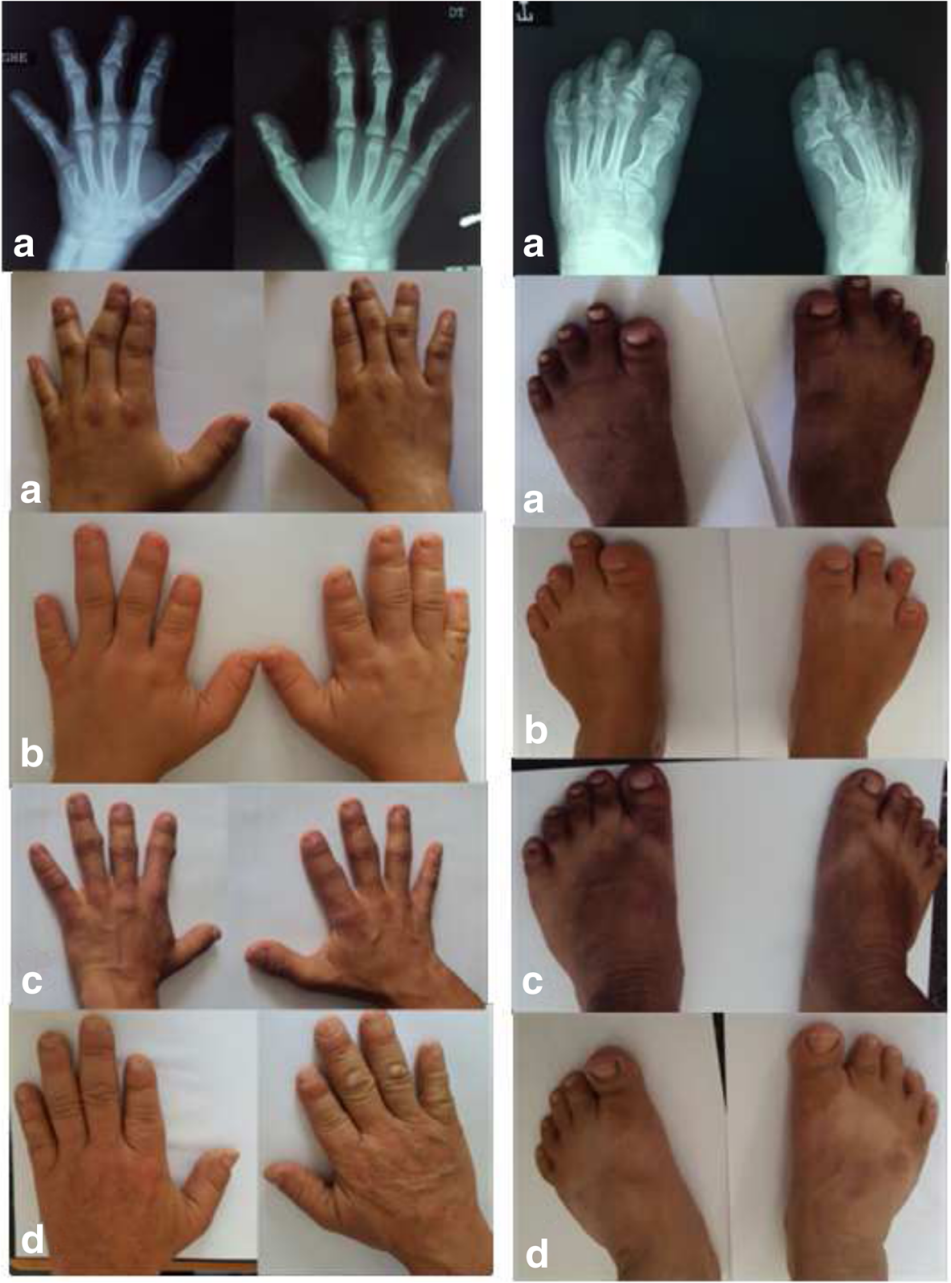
Characteristic hands and feet disclosing severe brachydactyly with syndactyly in the family members. a- Proband, b- Sister, c- Brother, d- Father. X-rays of the proband show misalignment of the middle and distal phalanges predominant at the third and fourth fingers with cone shaped epiphyses in hands and feet
